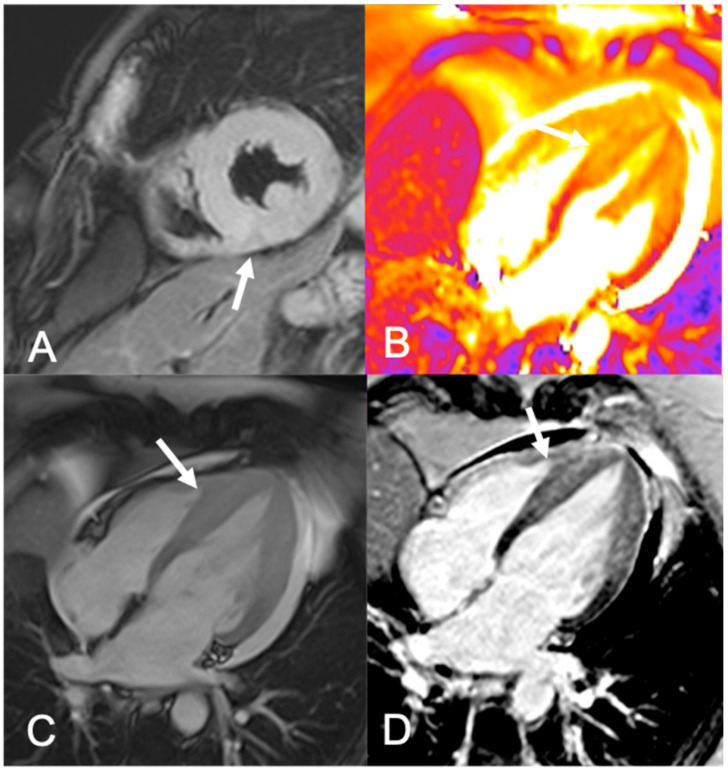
A 55-year-old woman on suspicion of Takotsubo syndrome. Coronarography showed no coronary stenosis. (A) T2-weighted short-axis image showing diffuse edema of the left ventricle middle–apical segments (arrow). (B) T2 mapping four-chamber view confirming the presence of edema (arrow). (C) Cine four-chamber TRUFI image showing thickening (arrow) of mid-apical segments of the left ventricle and hypokinesia. (D) In the four-chamber image, there was no appreciable ischemic pattern of LGE (arrow).
